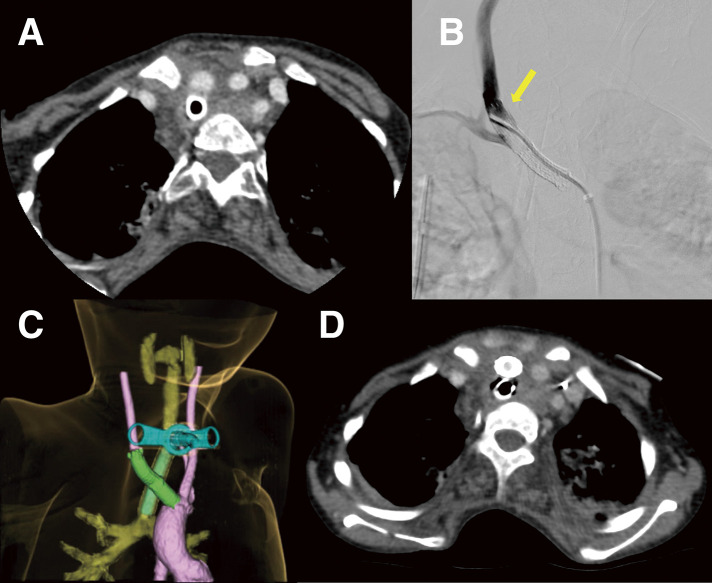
Representative imaging of a pediatric TIF from diagnosis to early postoperative assessment. (A) Axial contrast-enhanced computed tomography showed close apposition between the innominate artery and the trachea at the level of the tracheostomy tube cuff, without evidence of pseudoaneurysm formation or contrast extravasation. (B) Postoperative angiography at the distal edge of the VIABAHN stent graft (W. L. Gore & Associates, Flagstaff, AZ, USA) demonstrated a small type Ib endoleak (arrow). (C) Postoperative computed tomography on day 3 showed patent stent grafts without endoleaks. The proximal end of the VIABAHN stent graft extended slightly into the aortic arch. (D) An axial image demonstrated no contrast leakage between the stent graft and the native vessel wall, confirming complete exclusion of the fistula. TIF: tracheo-innominate artery fistula
